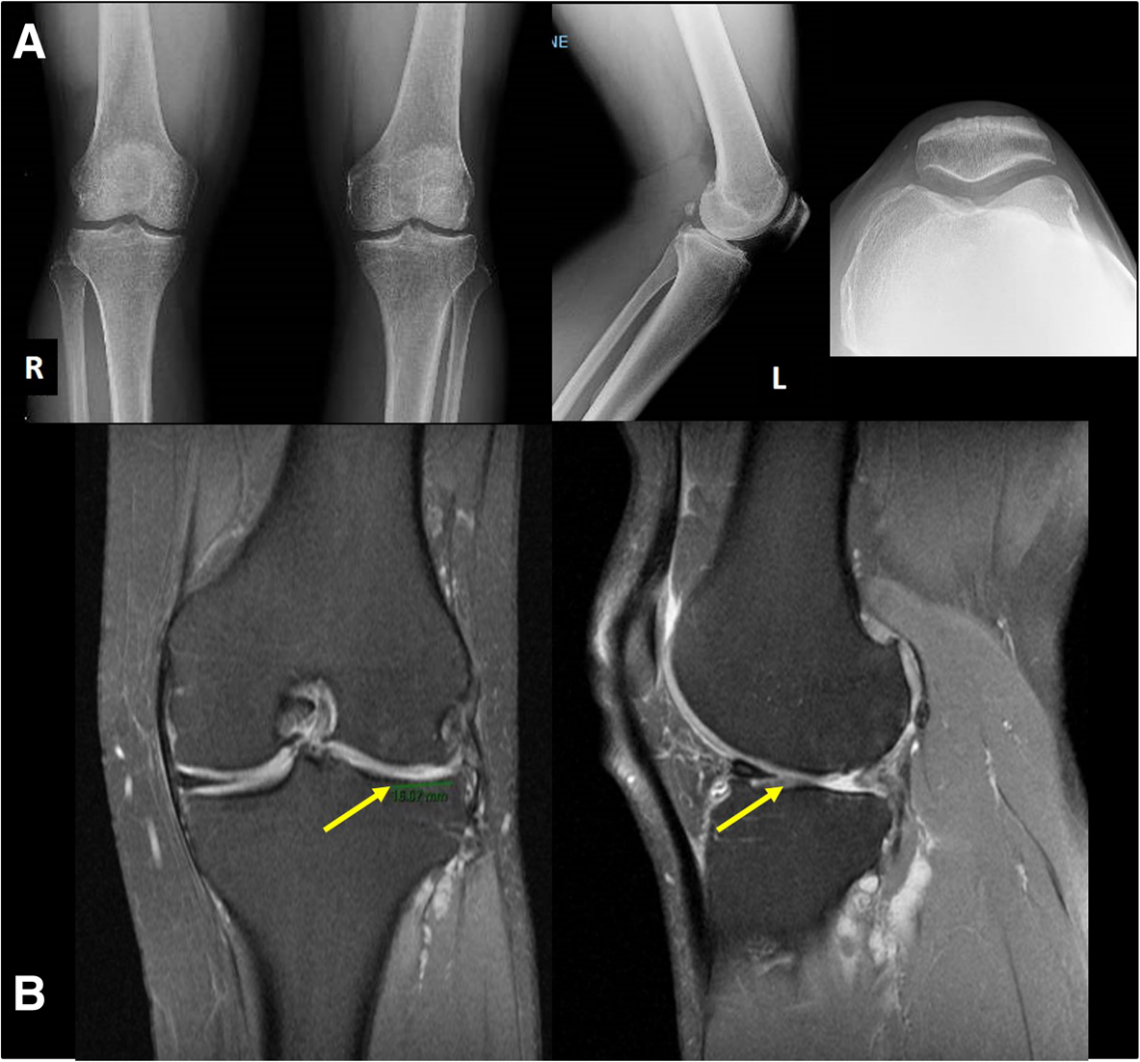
A 46-year-old man, with a grade IV cartilage injury of the lateral condyle and tibial plateau. a Preoperative plain radiographs. b Preoperative MRI showing a grade IV cartilage injury of the lateral condyle (30 × 20 mm) and grade III–IV cartilage injury of the tibial plateau (20 × 20 mm). Microfractures have been performed at the tibial plateau. The wider defect of the femoral condyle has been repaired with the Chondro-Gide® scaffold and BM-MSCs differentiated to chondrocytes
